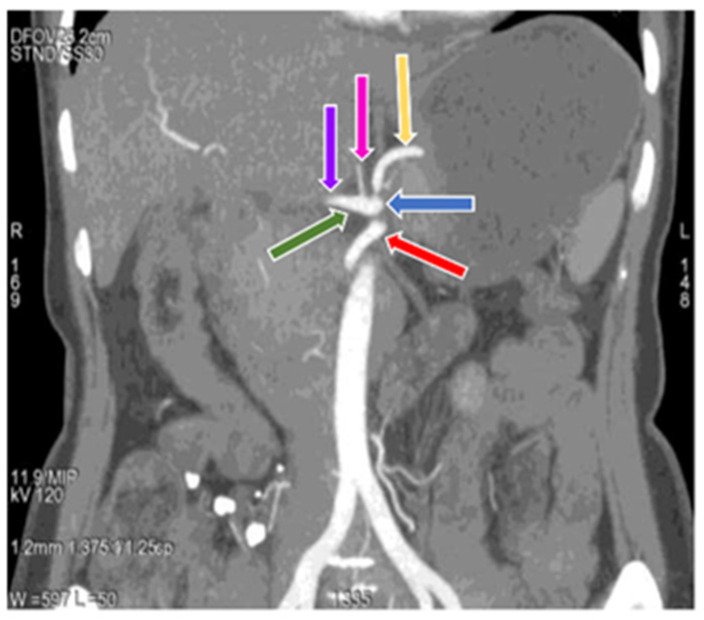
Coronal maximum intensity projection (MIP) image of (64 row scanner CT angiography) abdominal region showing superior mesenteric artery (red arrow) and division of celiac trunk (blue arrow) into gastro-hepatic trunk (dark green arrow) and splenic artery (yellow arrow), gastro-hepatic trunk further divides into common hepatic artery (purple arrow) and left gastric artery (pink arrow). Slice thickness: 1.2 mm.
